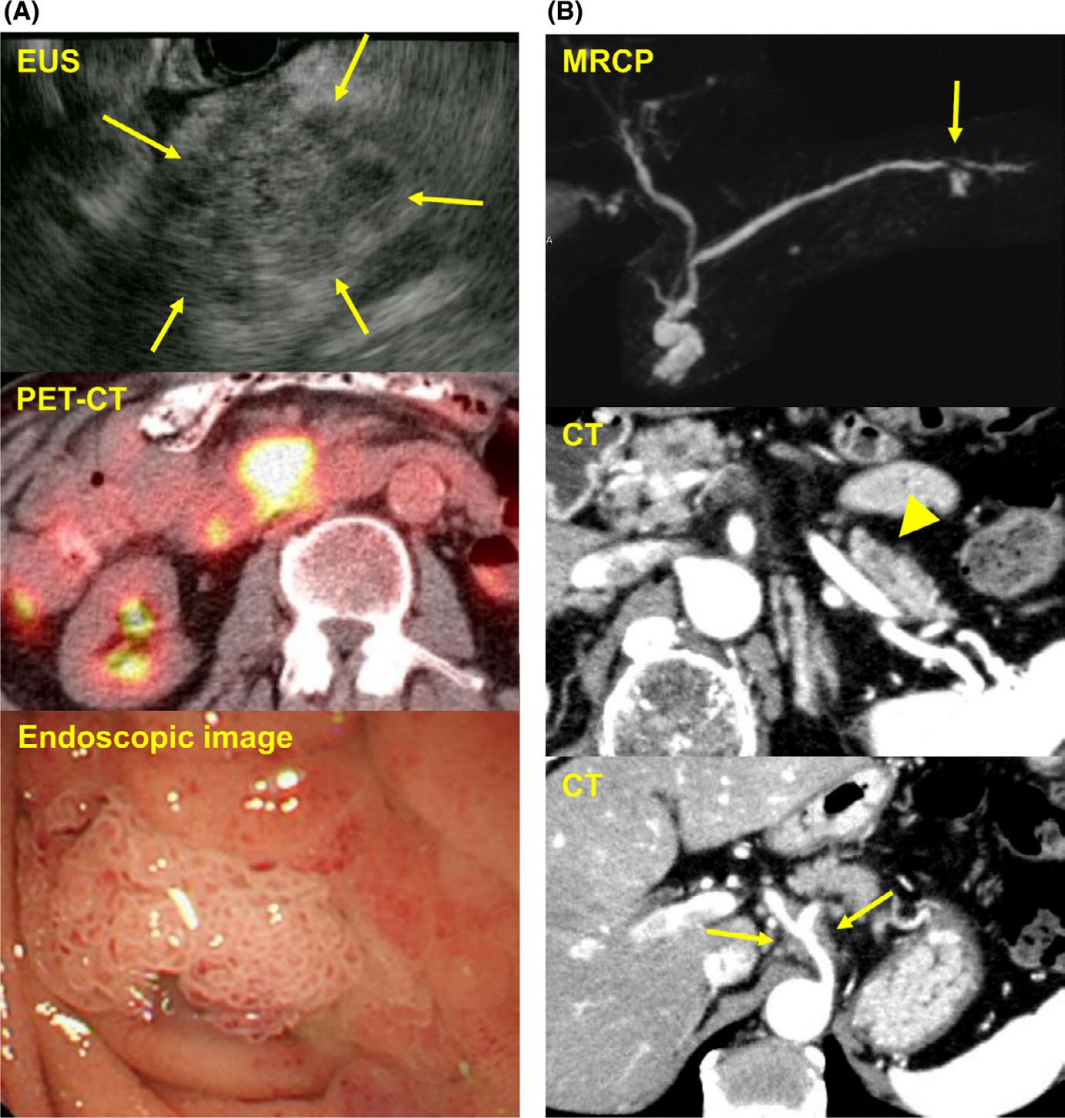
Clinical images of cases with a high number of mutations together with the *GNAS* mutation. Clinical images of cases with the GNAS mutation in Table 3 are shown. (a) Case 3 in Table 3. Positron Emission Tomography (PET)‐CT shows the accumulation of injected agents in the pancreas head in which a hypoechoic tumor with cystic lesions was observed by endoscopic ultrasonography (EUS, yellow arrows). Endoscopic image showing that the tumor invaded the duodenal lumen. (b) Case 4 in Table 3. Magnetic resonance cholangiopancreatography (MRCP) shows cystic lesions in the pancreas head and tail (yellow arrow). CT shows a hypo‐enhanced mass with a cystic lesion in the pancreas tail (yellow arrowhead) and shows the tumor extending along the arteries (yellow arrow)
